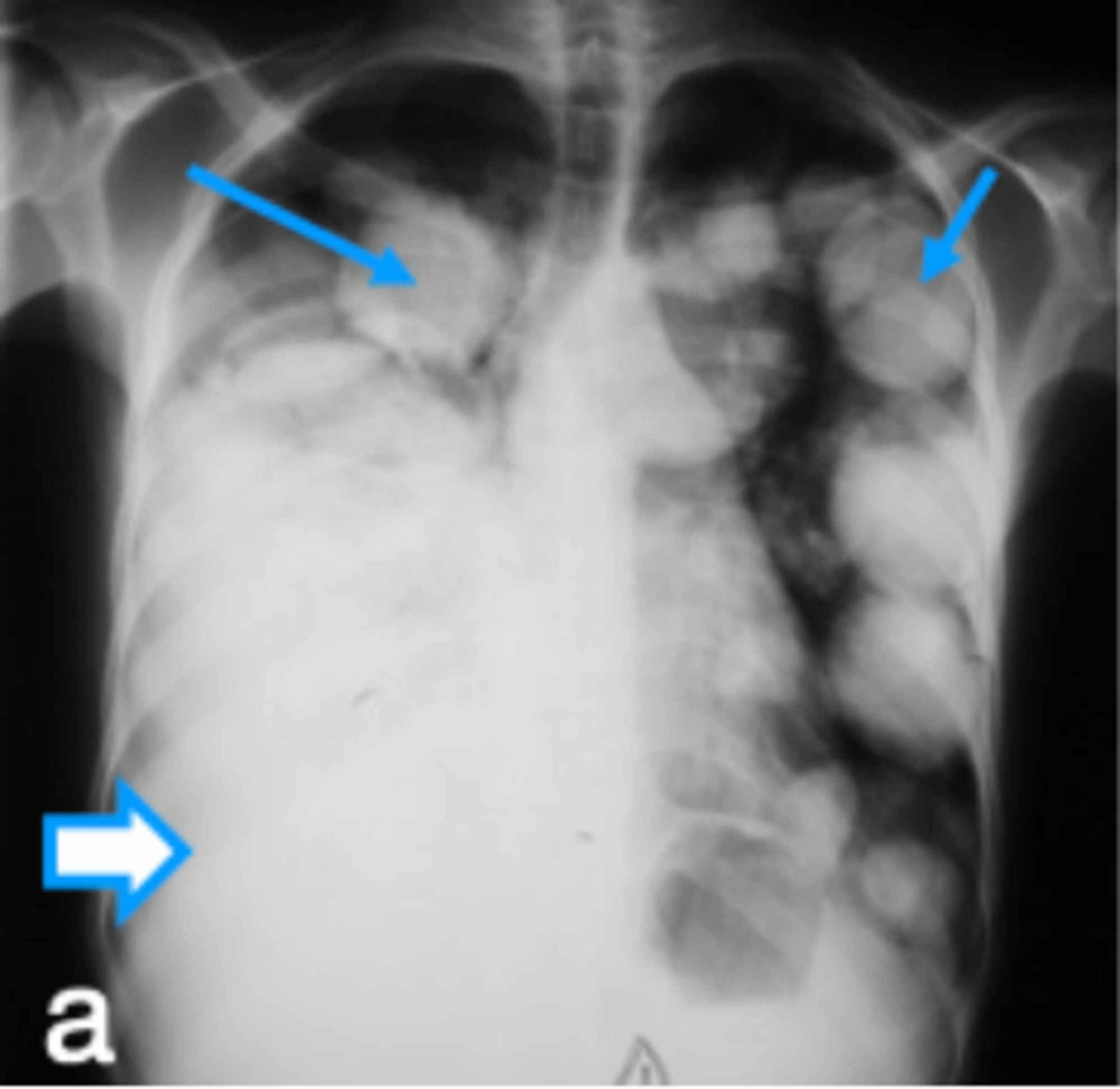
CXR frontal views before drainage of right pleural effusion, revealing multiple bilateral nodules and masses of varying sizes Thin arrows indicate echinococcal nodules and thick arrow indicates right pleural effusion. CXR: chest X-ray
